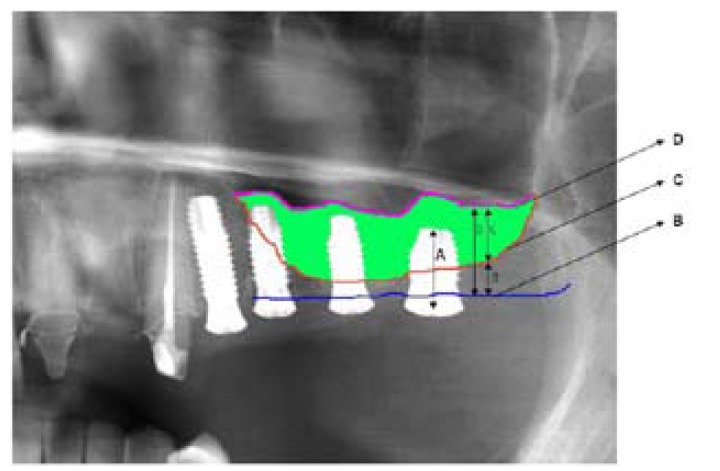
Postoperative panoramic X-ray view. Measurement of bone gain.
